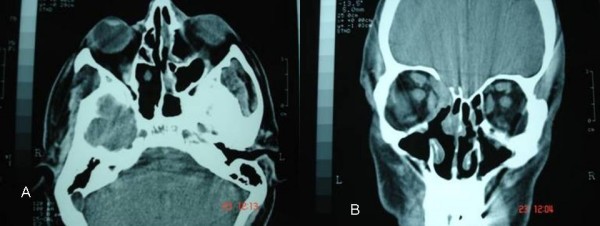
Postoperative computed tomographs a) axial and b) coronal.
